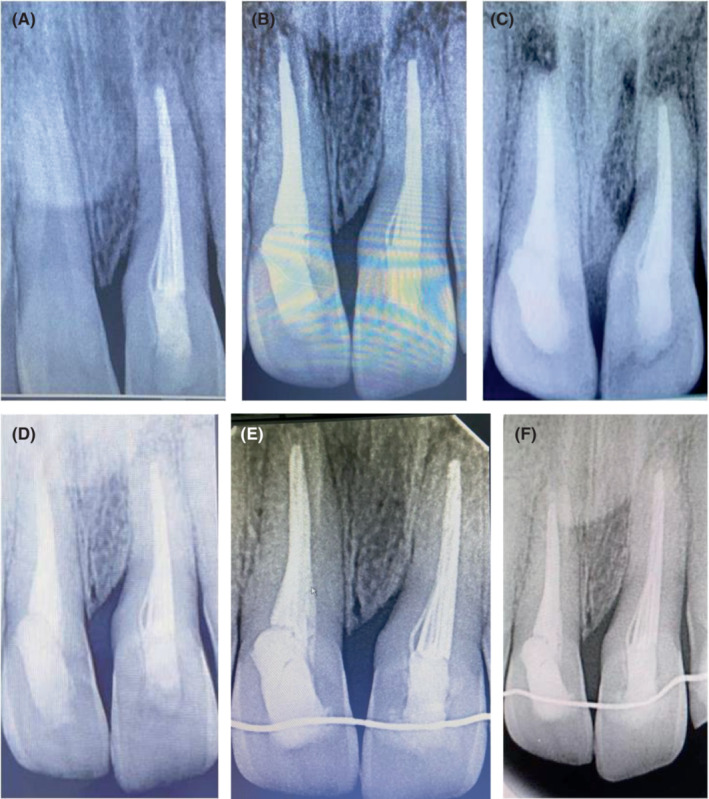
Radiographs of Follow‐up—(A) 6‐month (B) 1‐year (C) 2‐year (D) 3‐year (E)4‐year (F) 5‐year.
